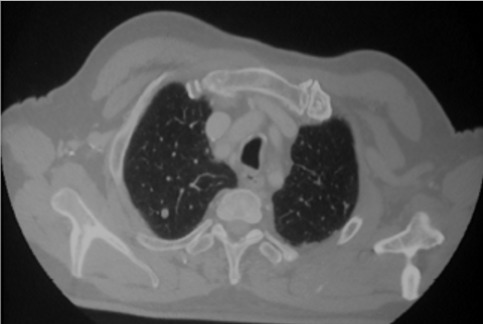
Axial CT scan in parenchymal window showing wright apicalpulmonary nodule of 6 mm
